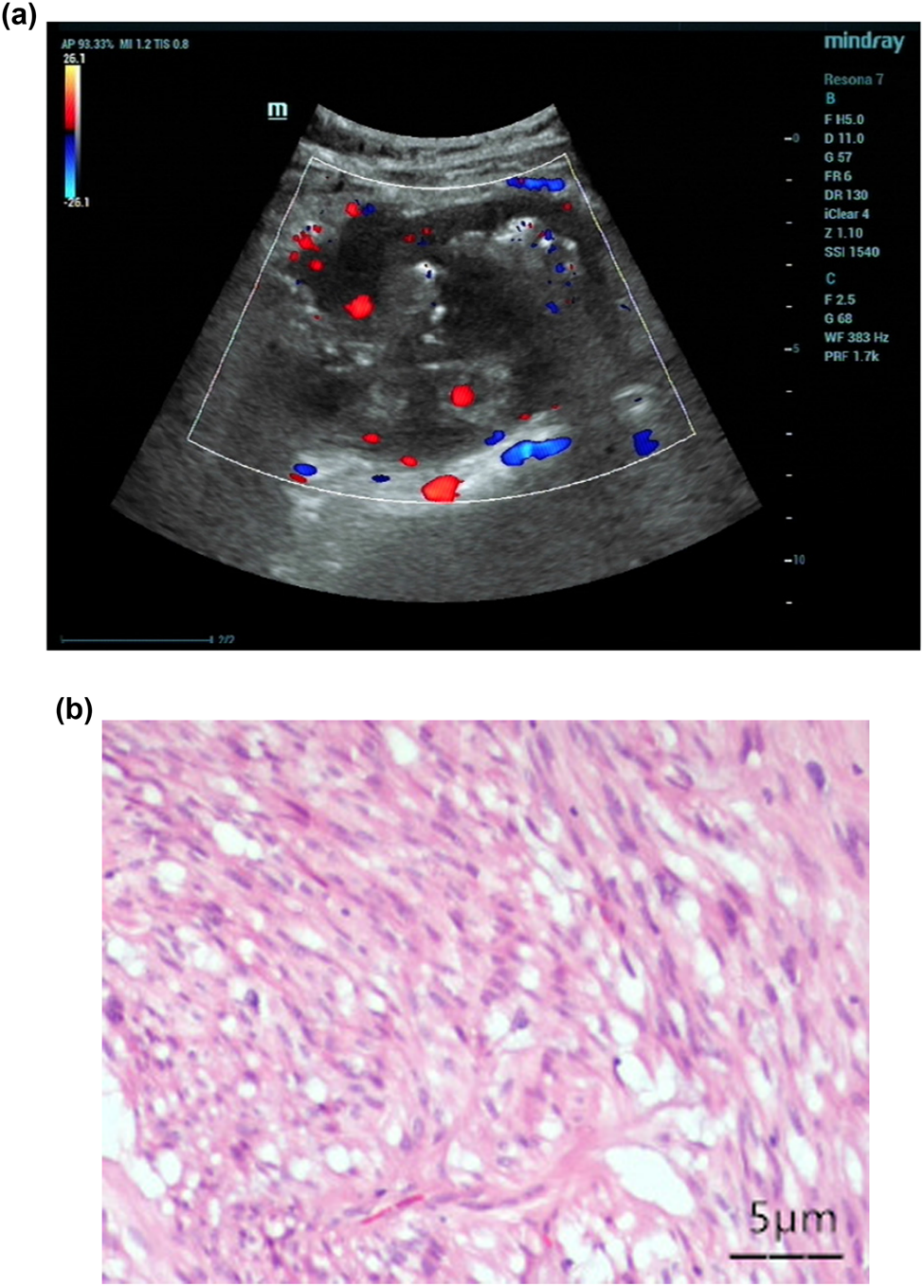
(a) Preoperative color Doppler ultrasonography in a GST patient: solid lesion in the right mid-upper abdomen (below the lower pole of the right kidney and above the right side of the inferior vena cava). (b) Postoperative pathological findings of a GST patient: high-risk gastrointestinal stromal tumor (multiple masses with visible necrosis and vascular invasion).
